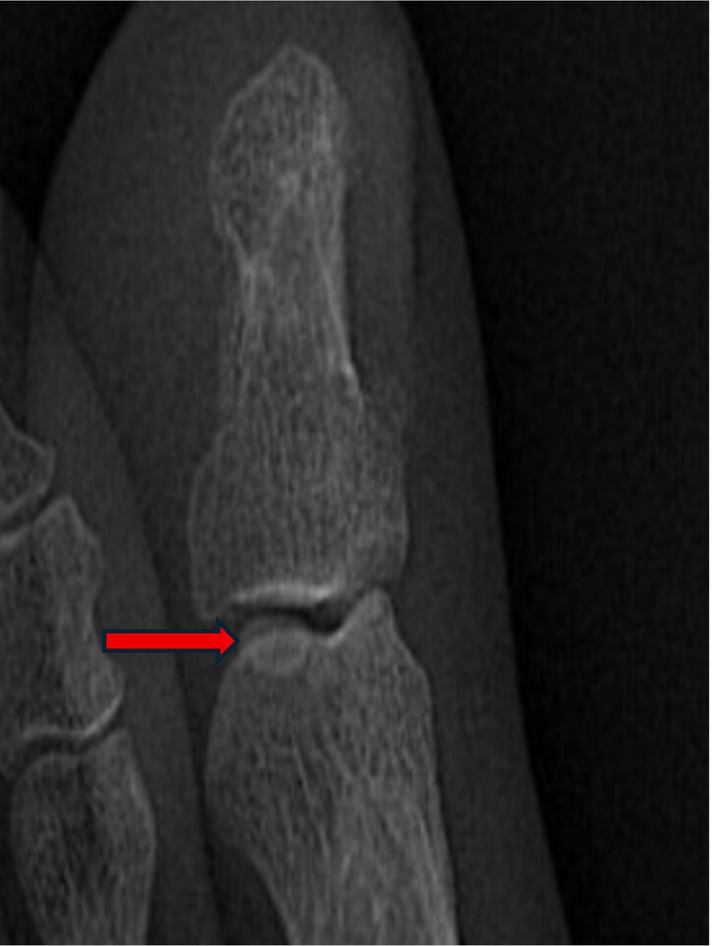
Magnified radiographic imaging of the left foot clearly reveals a sesamoid bone within the interphalangeal joint. The structure indicated by the red arrow corresponds to this sesamoid bone.
